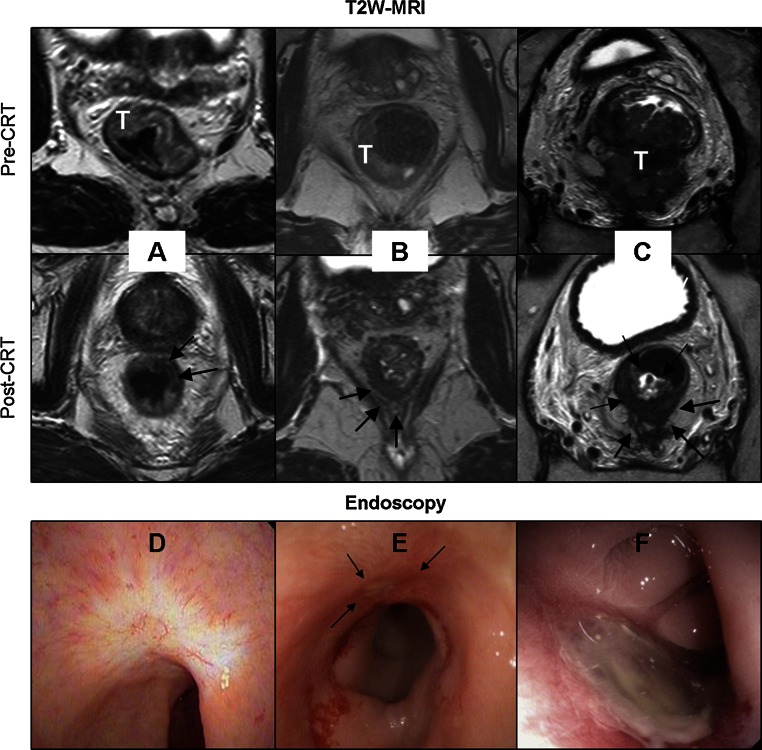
Response assessment with T2W-MRI (a–c) and with endoscopy (d–f). Pre- and post-CRT MR images are shown. T indicates tumor; arrows indicate scar or residual tumor after CRT. a Typical CR at T2W-MRI, b equivocal image at T2W-MRI, and c obvious residual tumor at T2W-MRI. d Typical endoluminal image of CR with white scar with teleangiectasia. e Small ulcer with smooth edges (*arrows*) but without residual polypoid tissue. Patients imaged in (d) and (e) experienced sustained clinical CR at follow-up. f Example of large ulcer that was deemed residual tumor after CRT
